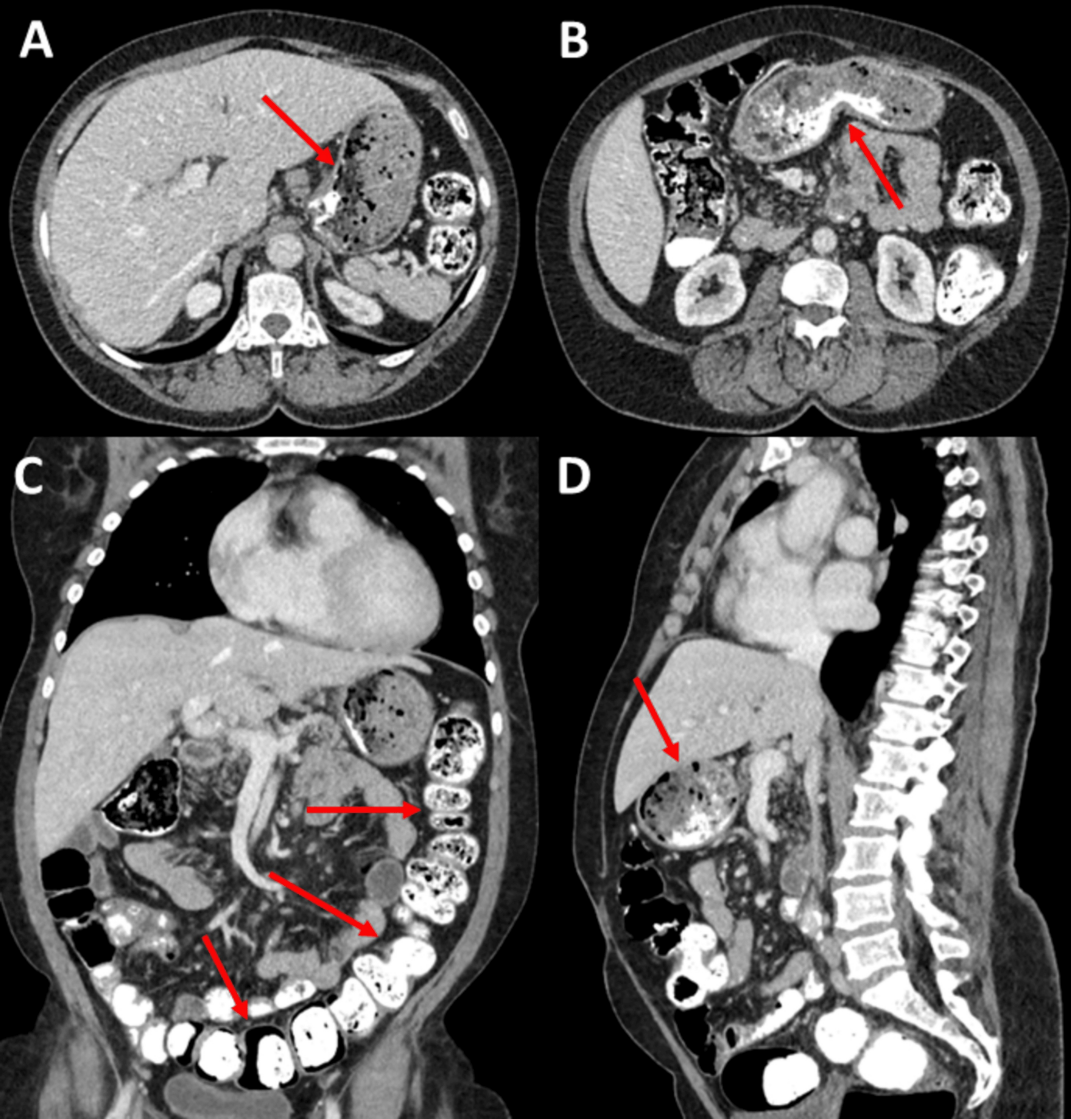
Abdominal and Thoracic CT Scan Axial (A, B), coronal (C), and sagittal (D) CT images demonstrate high-density material in the gastrointestinal tract (arrows), consistent with sand ingestion related to pica behavior. The images highlight the presence of radiopaque content within the stomach and intestines, which correlates with the patient's history of ingesting sand during periods of stress or anxiety.
